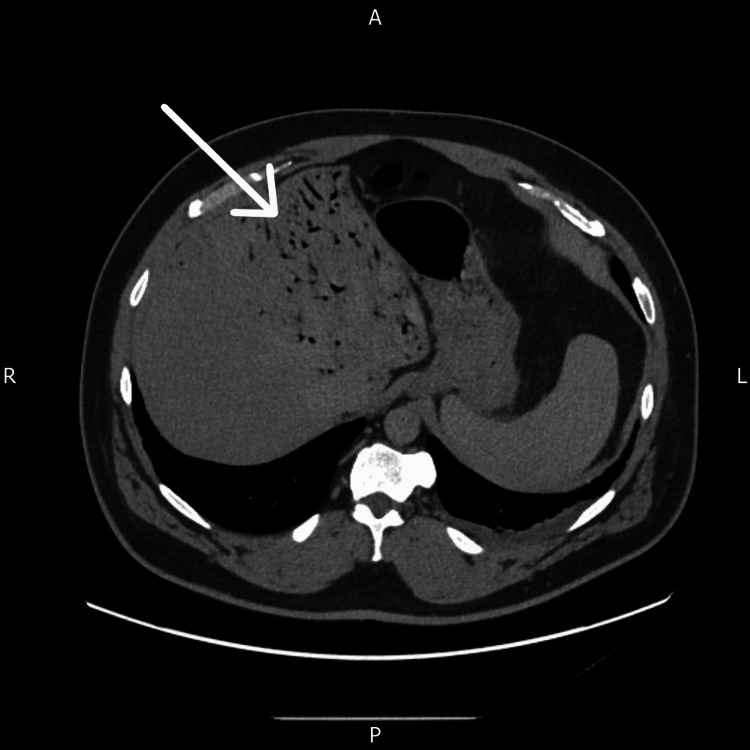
Repeat axial CT scan of the abdomen showing a reduced volume of portal venous gas within the left lobe of the liver
